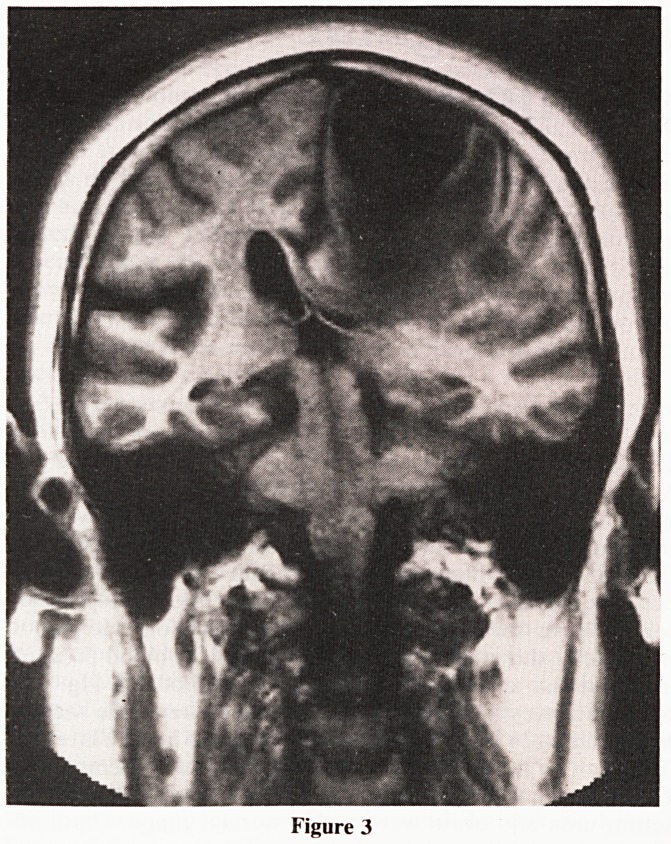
# Imaging of Intracranial Tumours—Comparison of Computed Tomography and Magnetic Resonance Imaging
